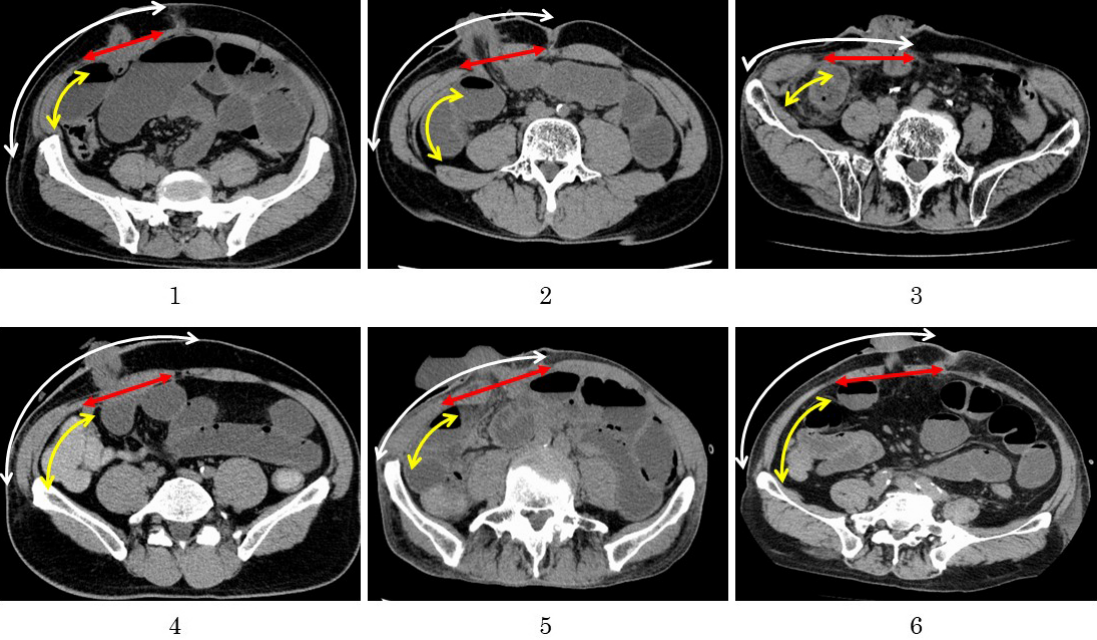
CT images of the six patients who have developed SOO. The transverse abdominal muscle and the internal and external oblique muscles extended laterally, but the abdominal rectus muscle did not under pneumoperitoneum. Thus, the skin and subcutaneous layer were displaced laterally from the muscular layer. Yellow arrow: transverse abdominal muscle and internal and external oblique muscles, red arrow: abdominal rectus muscle, white arrow: skin and subcutaneous layer.
